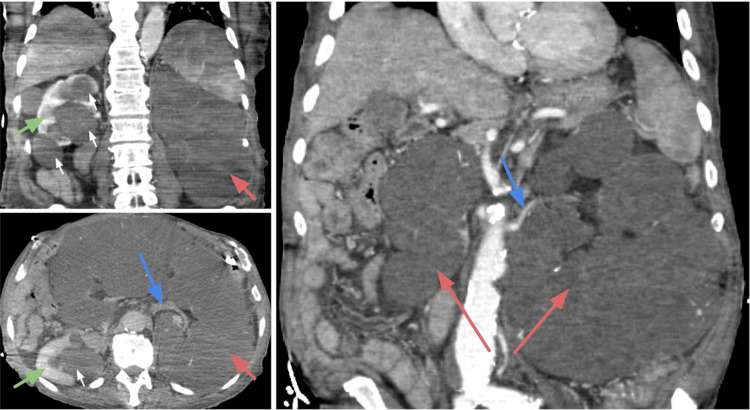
Abdominal CT scan with intravenous contrast. Red arrow: left kidney with cystic changes; blue arrow: left renal artery; green arrow: right kidney; white small arrows: cysts.
